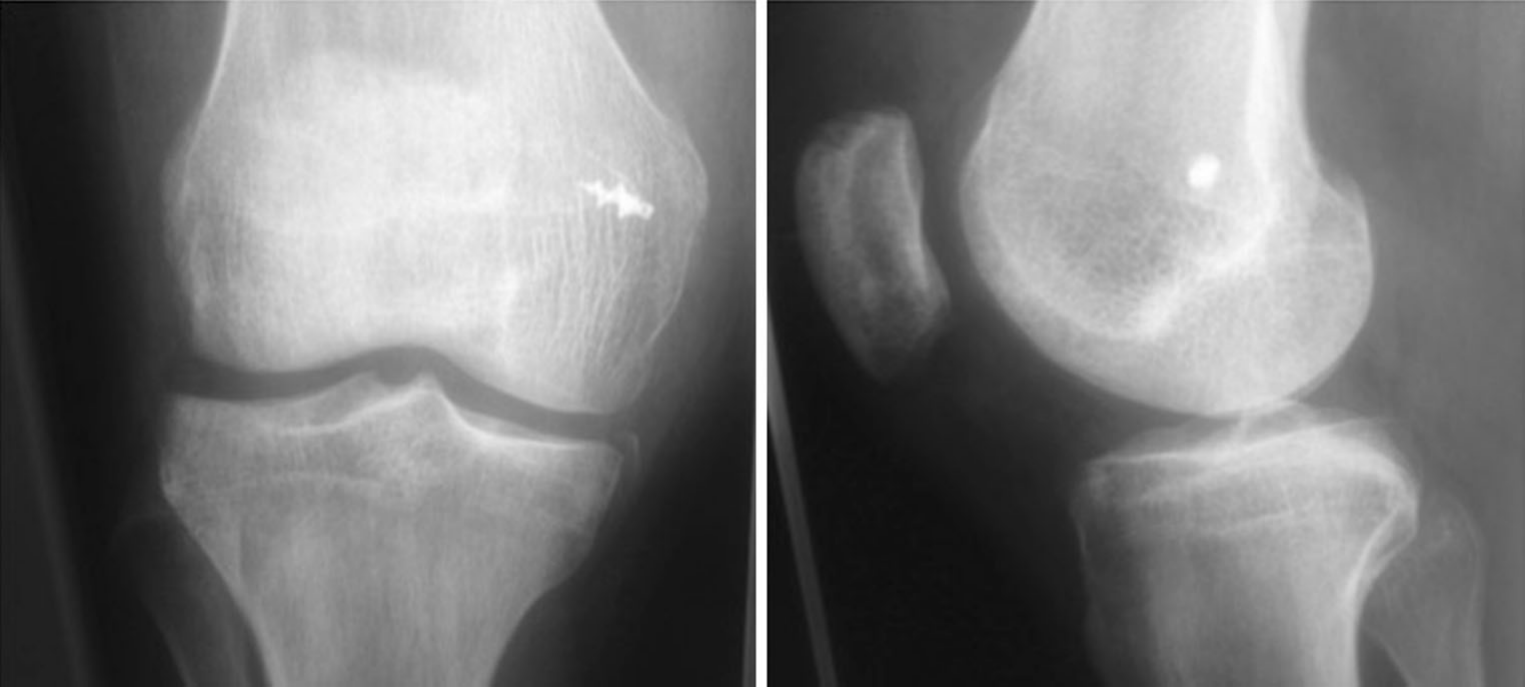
Postoperative radiograph showing the correct insertion of the suture anchor at the isometric femoral point as described by Steensen et al.
